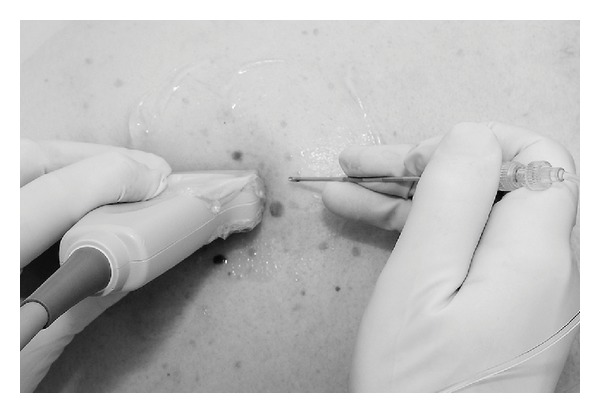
Thoracic paravertebral block technique using a 16 G Tuohy needle with a craniocaudal in-plane technique and a high frequency ultrasound transducer.
